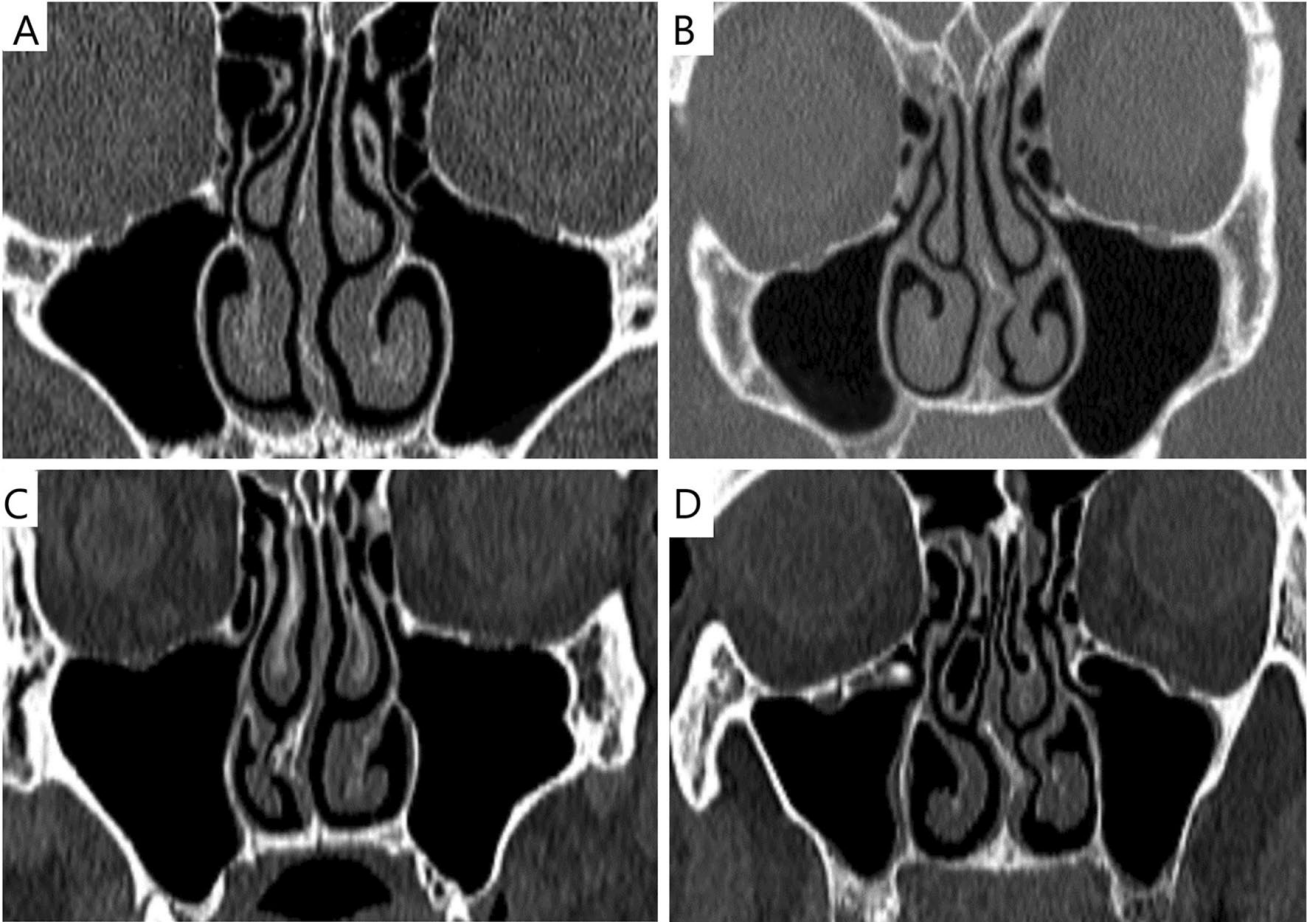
CT scans, frontal projection of the nasal sinuses. Maxillary sinus ostium level. (A) Patient 1 (norma); (B) Patient 2 (NSD in the left side and NTH); (C) Patient 3 (NSD in the right side); (D) Patient 4 (NSD in both side and BCB).
